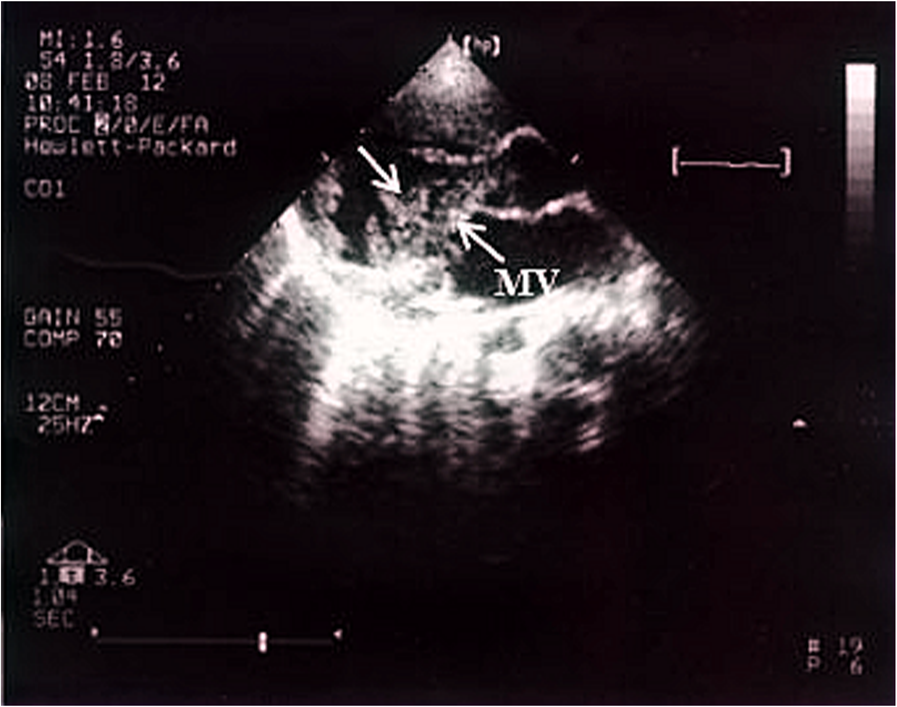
Preoperative echocardiography image of the CPFE adherent to the mitral valve. MV: mitral valve.
